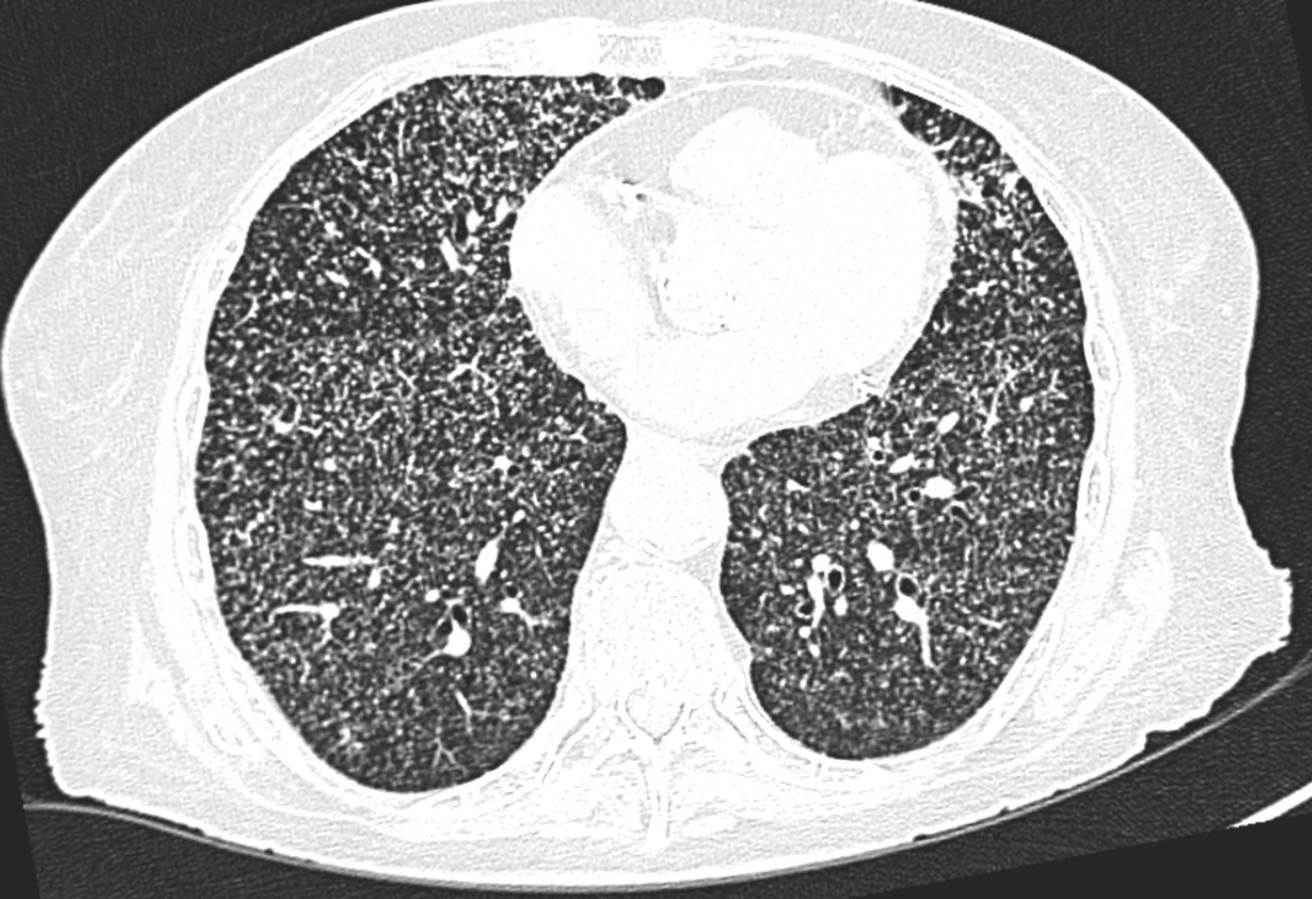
Axial high-resolution computed tomography (HRCT). Innumerable, randomly distributed micronodules (1-3 mm) throughout both lungs, predominantly in the middle and lower zones, consistent with miliary dissemination.
